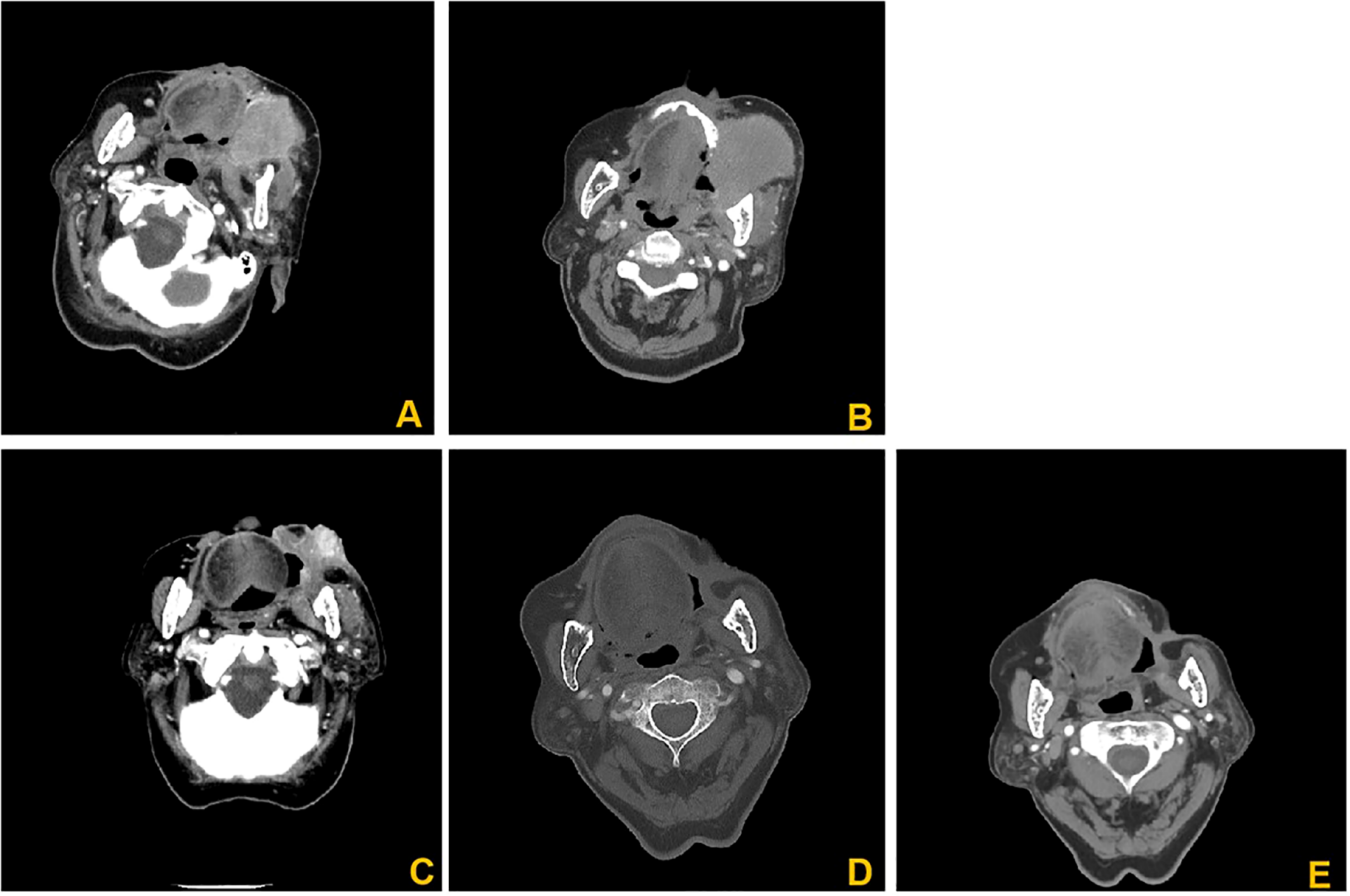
CT images of an 86-year-old female patients. (A) Enhanced CT images before interventional treatment; (B) Enhanced CT showed significant low-density changes of the tumor 7 days after treatment; (C) Enhanced CT showed that the lesion was significantly reduced, and no significant enhancement was found 1 months after treatment. (D) 3 months after treatment, the lesions basically disappeared; (E) 6 months after treatment, the features of the lesion were basically the same as those in D, without significant changes.
